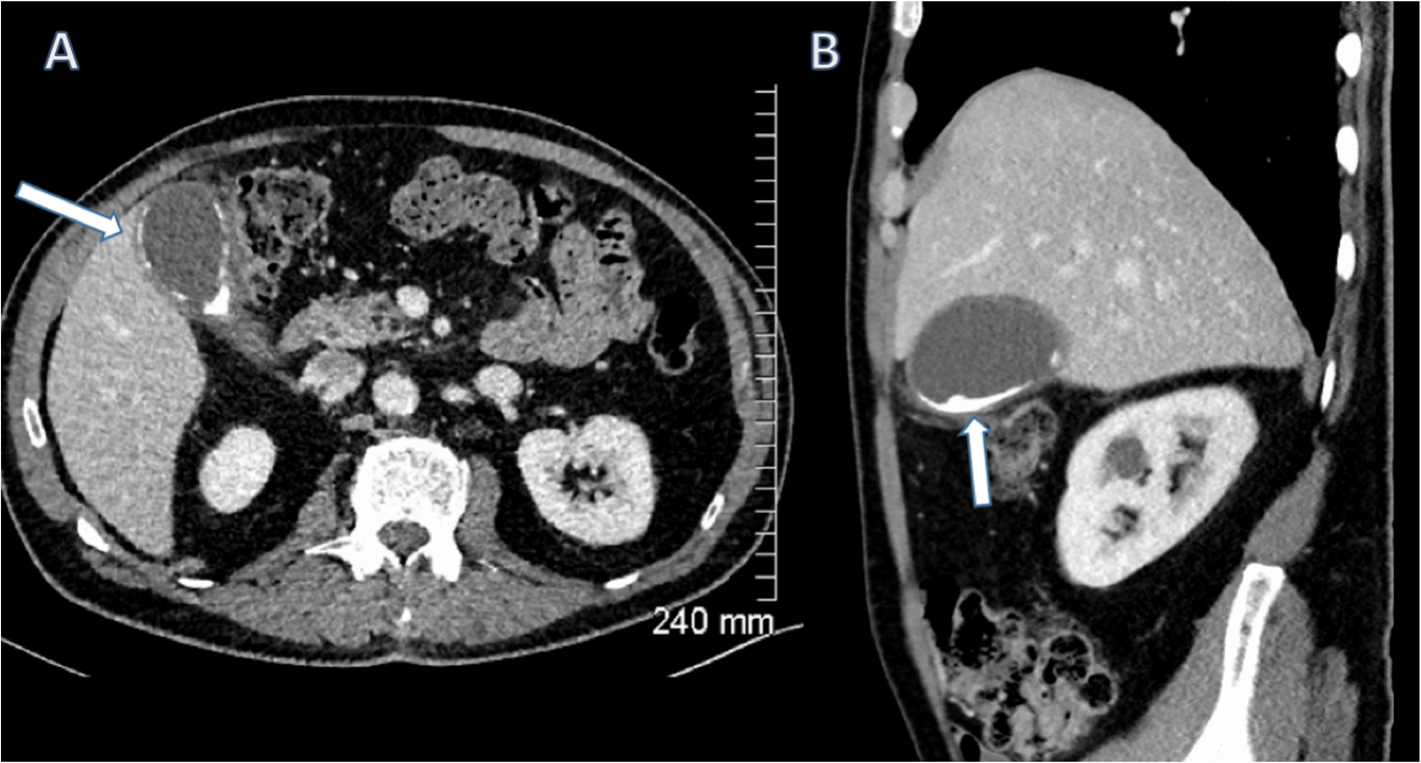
Axial (a) and sagittal (b) contrast-enhanced CT of the abdomen demonstrating peripheral calcification of the gallbladder wall (arrows) consistent with porcelain gallbladder
